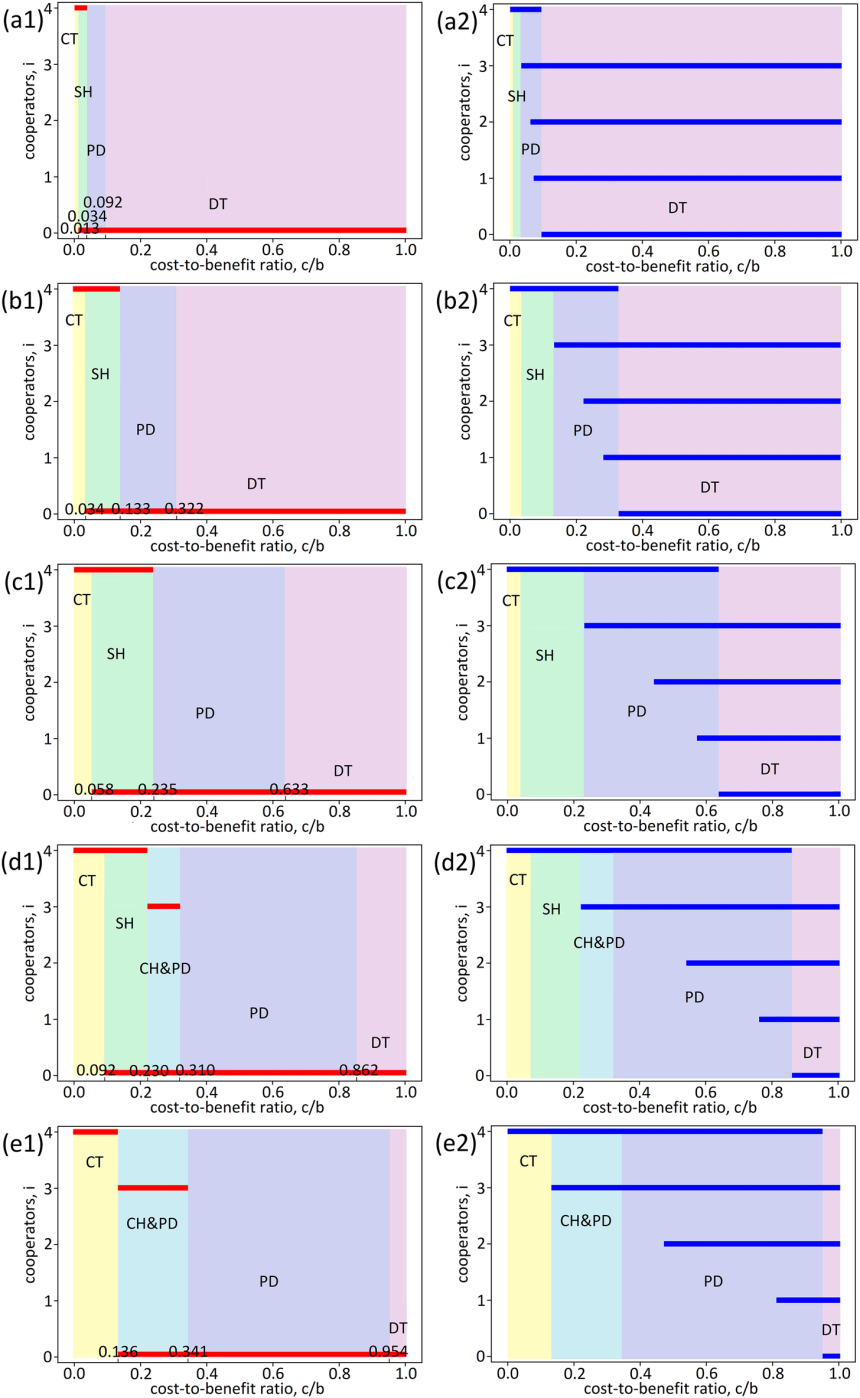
Equilibria and optimal strategies of the four-player weightlifting game. Nash equilibria (a1,b1,c1,d1,e1) and Pareto optimal strategies (a2,b2,c2,d2,e2). (a1,a2) $$\mu =10$$. (b1,b2) $$\mu =20$$. (c1,c2) $$\mu =30$$. (d1,d2) $$\mu =40$$. (e1,e2) $$\mu =50$$. The parameter regions for Nash equilibria and Pareto optimal strategies are as hatched in the *i*-$$c/b$$ plane, where *i* is the number of cooperators and $$c/b$$ is the cost-to-benefit ratio. We set $$\sigma =50$$ in all cases. All players cooperate for a small value of $$c/b$$ (CT), while they defect for a large value (DT).
